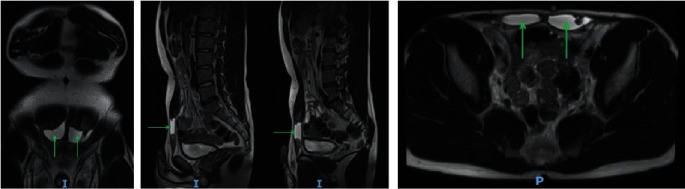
Coronal, sagittal, and transverse magnetic resonance images of the patient, arrows highlight the bilateral defect in the rectus muscles. I, inferior and P, posterior.
